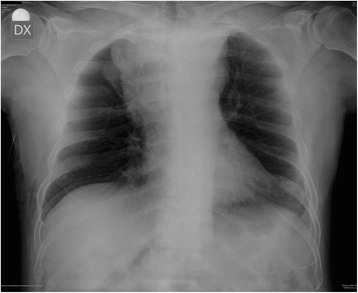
Chest X-ray; antero-posterior view. Upper mediastinal widening due to a mediastinal mass (10 cm × 6 cm), more evident on the right side, with polycyclic margins; the angles with the mediastinal contour are obtuse. The hilar vessels cannot be seen through the mass (“hilum overlay sign” absent). The paravertebral line can be recognized. These two findings confirm that this mass is located in the superior-anterior-middle mediastinum. Trachea is deviated laterally to the right side by the mediastinal mass. At the right inferior chest wall subcutaneous tissue mass can be detected
